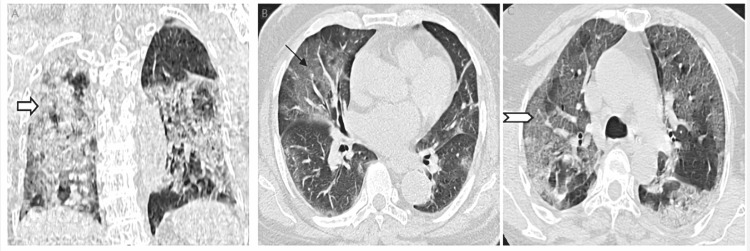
HRCT images of patients classified in severe category. A: Coronal image showing diffuse GGOs involving bilateral lung parenchyma with consolidatory changes (severe category). Block arrow showing diffuse involvement of lung parenchyma with ground glass opacities, consolidatory, and fibrotic changes. B: Axial image showing GGOs involving bilateral lung parenchyma (severe category). Arrow showing diffuse GGO. C: Axial image showing GGOs with surrounding consolidatory changes (severe category). Chevron shows diffuse GGOs and consolidatory changes. Note diffuse involvement of lung parenchyma in the severe category. HRCT, high resolution computed tomography; GGO, ground glass opacity
